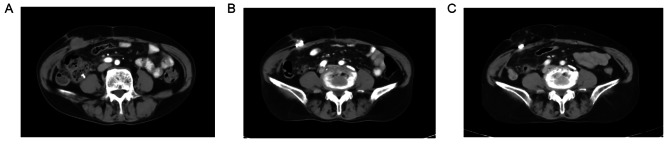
Images from a 68-year-old patient with S-STM of colorectal cancer. (A) Preoperative CT enhanced scanning images showed an abdominal wall lesion. (B) Two months after RISI, the lesions have shrunk significantly. (C) Six months after RISI, CR was achieved on the image and 125I seeds aggregation was left
